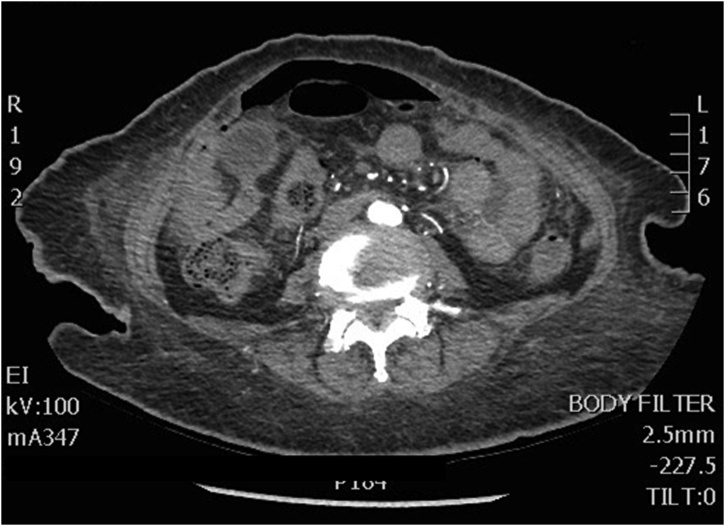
Plain Computed Tomography scan of the abdomen and pelvis showing moderate amount pneumoperitoneumwith thickening of the peritoneal reflections; consistent with viscous perforation.
